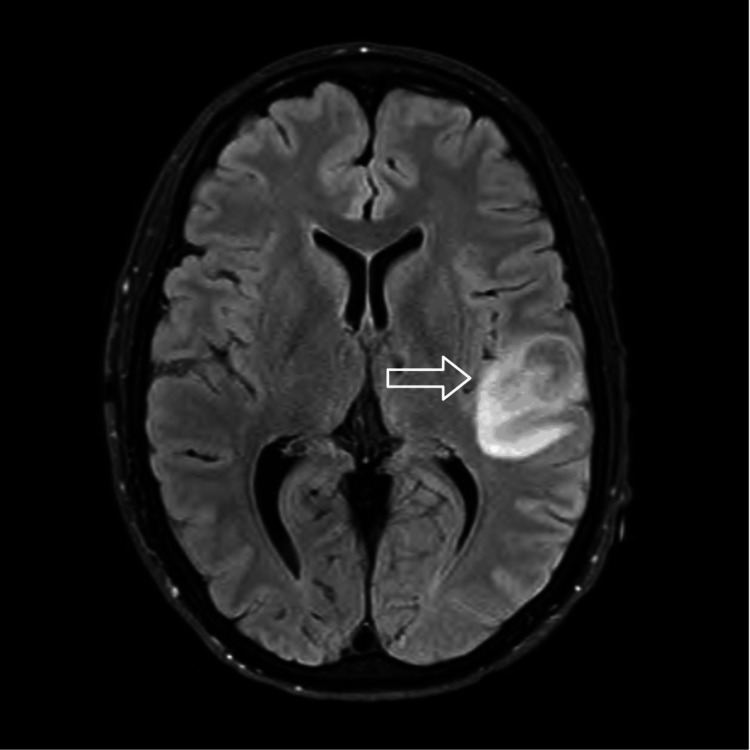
MRI brain scan with and without contrast with axial T2/FLAIR sequence revealing 17 x 20 mm round/oblong lesion with peripheral T2 hyperintensity centered around the superior temporal gyrus.
